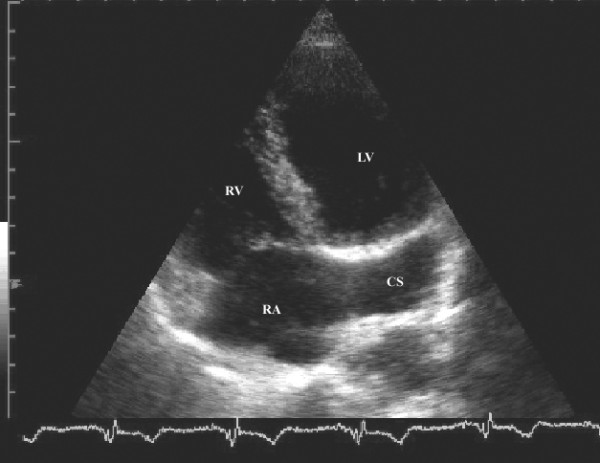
Modified apical 4 chamber two-dimensional echocardiographic view showing dilated coronary sinus with 2.8 cm in diameter. (LA = left atrium, LV = left ventricle, CS = coronary sinus)
